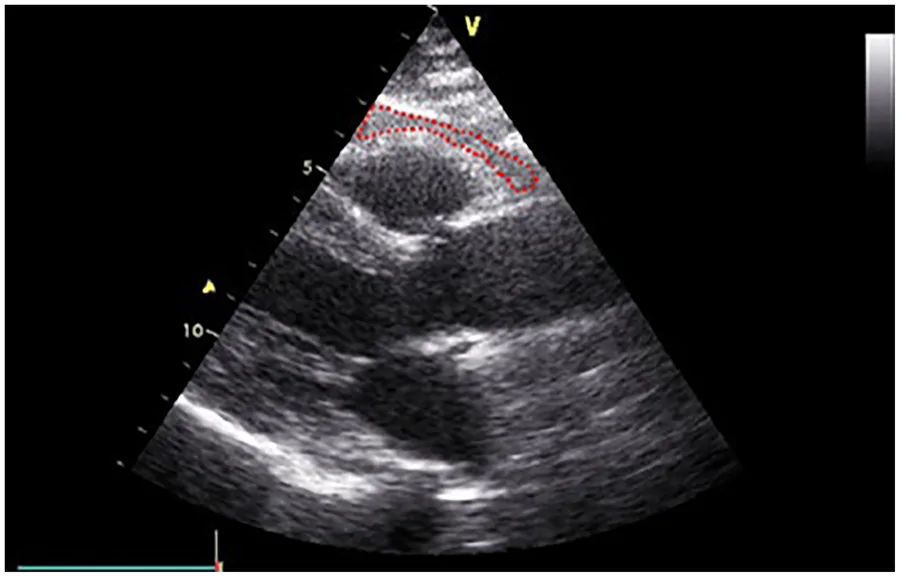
Echocardiographic measurement of epicardial fat thickness (EFT). Epicardial fat thickness was measured in the parasternal long-axis view as the echo-free space between the outer wall of the myocardium and the visceral pericardium overlying the free wall of the right ventricle. Measurements were obtained at end-systole, perpendicular to the right ventricular free wall, using the aortic annulus as an anatomical landmark.
